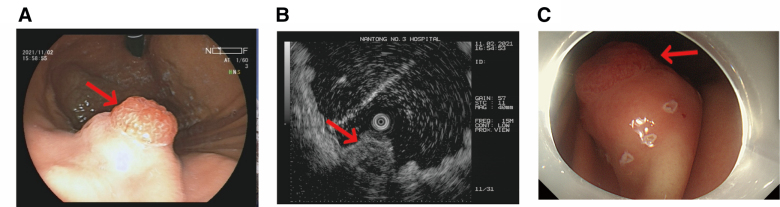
Image of GCP lesion gastroscopy. (A) Gastroscopy revealed a bulging lesion on the side of the greater curvature of the gastric body. (B) An ultrasonographic image from 2021 indicated that the lesion was located in the mucosal layer, and a duct-like echogenic area was observed underneath. (C) Marking of the lesional margins. GCP = gastritis cystica profunda.
